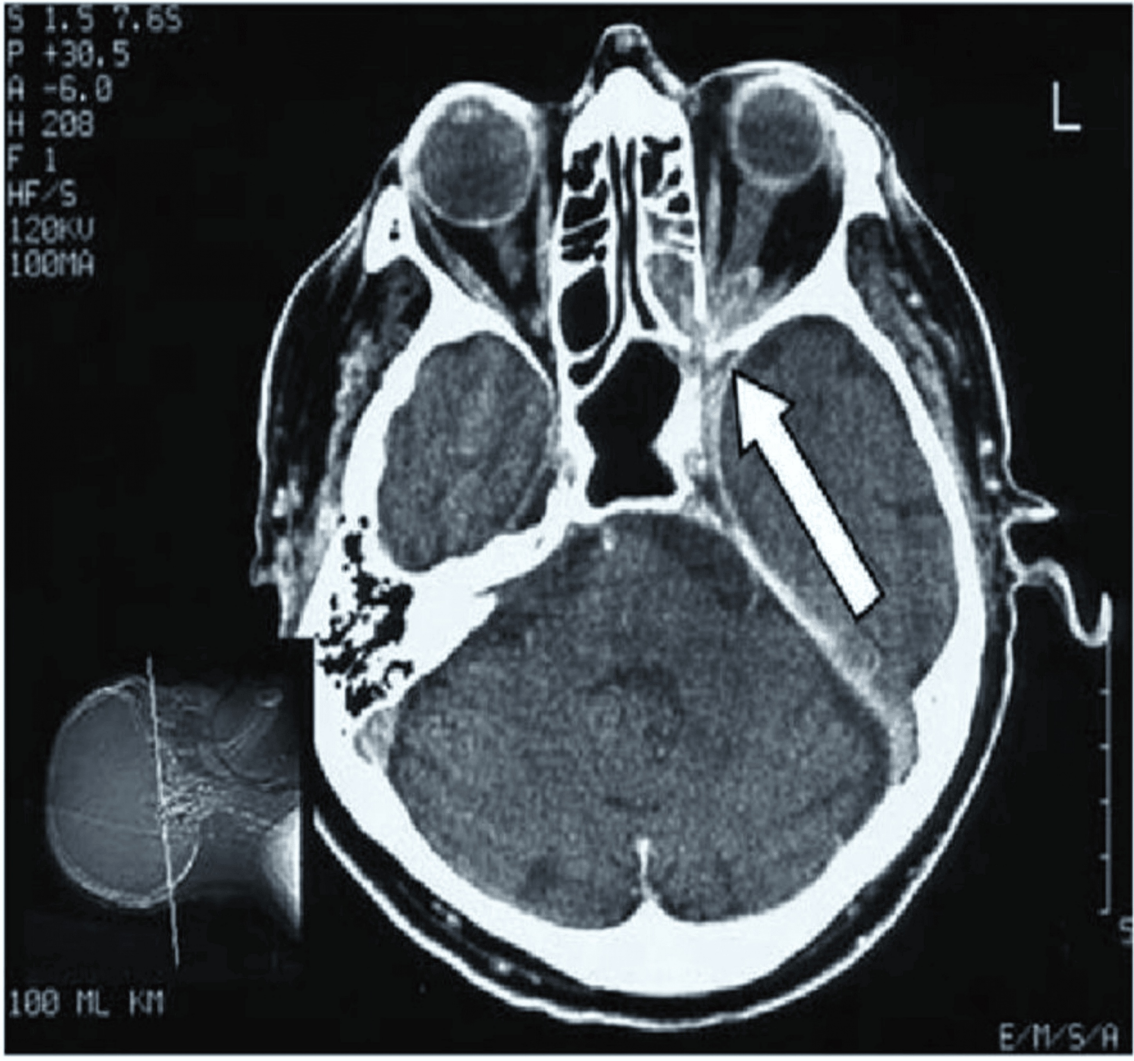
MRI brain with contrast of case 2. MRI with contrast demonstrated soft tissue opacification (arrow) in the sphenoid sinus with extension toward the orbital apex.
